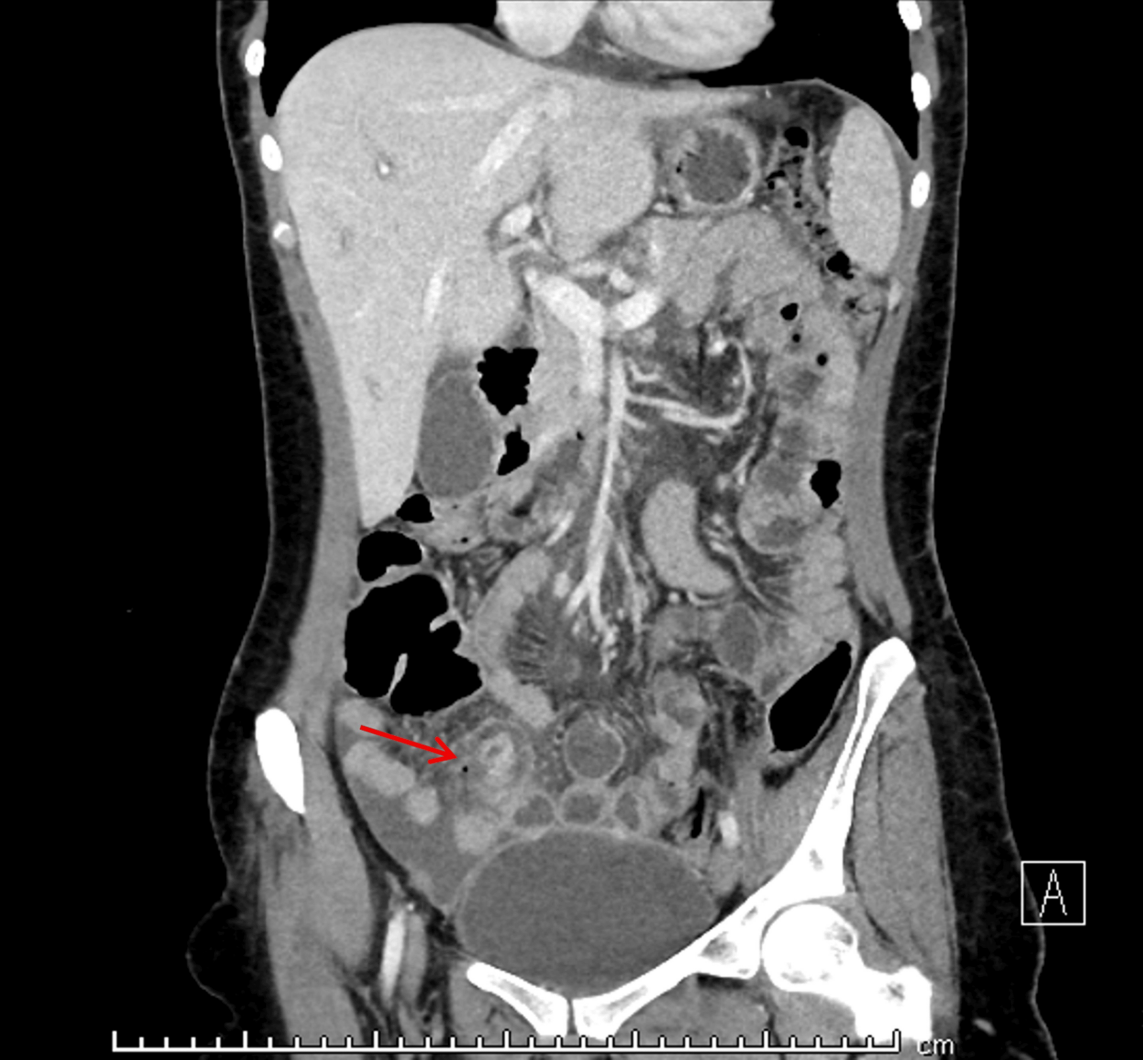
Coronal section contrast-enhanced CT showing perforated Meckel’s diverticulum (interrupted wall). The red arrow is pointing to a blind-ending, tubular structure arising from the distal ileum, with focal wall thickening and discontinuity, surrounded by fat stranding and inflammatory changes. This radiologic appearance is consistent with a perforated Meckel’s diverticulum.
